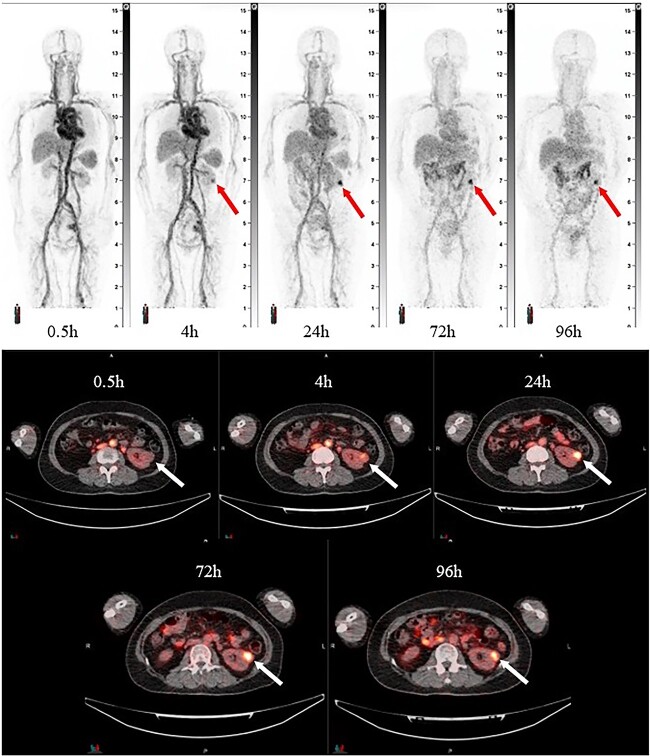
Representative [89Zr]Zr-DFO-girentuximab PET/CT images at 0.5, 4, 24, 72, and 96 h following injection. Top: maximum intensity projection images. Bottom: axial PET/CT fusion images. Arrows indicate the location of left renal lesion.
